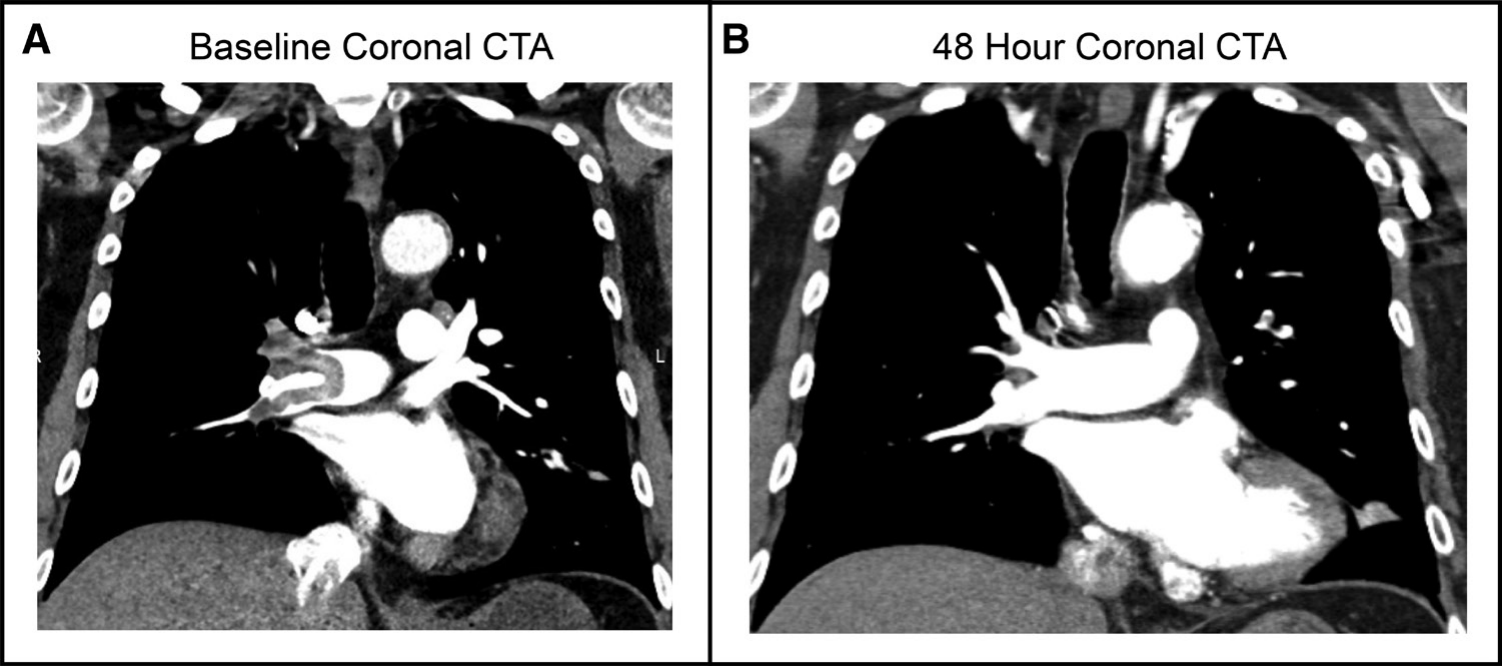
Case summary for a subject with right pulmonary artery embolism. A, Subject with predominantly right-sided pulmonary embolism graded as a refined modified Miller index of 19 at baseline. The subject was treated using a 24F Symphony catheter with an estimated aspirated blood volume of 400 mL and a substantial post-aspiration decrease in systolic pulmonary artery pressure (24-mm Hg reduction). B, Follow-up imaging demonstrated an 87% reduction in clot burden at 48 hours with a refined modified Miller index of 2.5 and a 46% decrease in right ventricle-to-left ventricle ratio from 2.03 to 1.09. The subject was discharged home later that day and experienced no adverse events through the 30-day follow-up. CTA indicates computed tomography angiogram.
